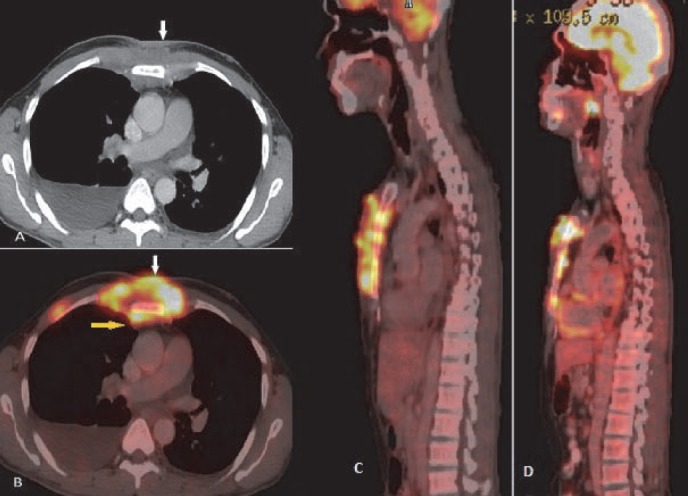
Lymphoma involving the chest wall. CT scan (A) and fused PET/CT images (B) show pre and parasternal ulceroproliferative plaque like lesion (arrow in A) with increased FDG uptake (white arrow in B). Deep extension of the lesion in retrosternal region at the middle 1/3^rd^ of the body of sternum is seen (yellow arrow in B). Post therapy scan shows (D) partial metabolic response as compared to pre therapy scan (C)
